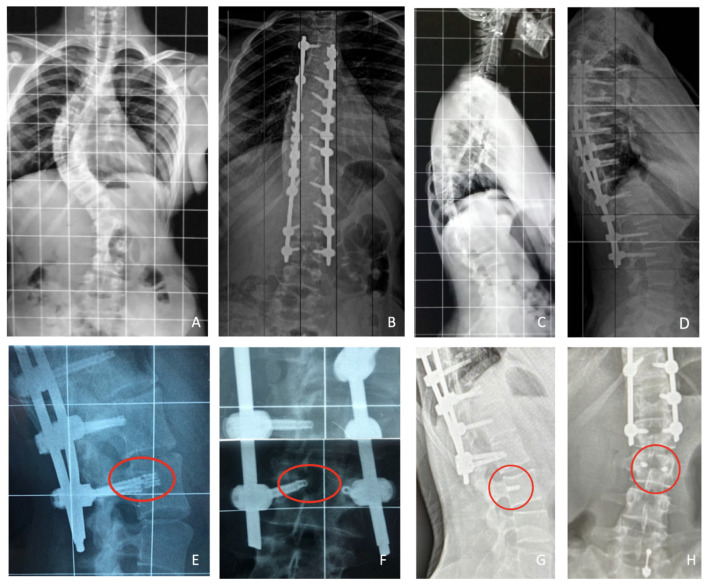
Coronal (A) and sagittal (C) standing X-rays of a 13-year-old AIS patient who underwent posterior T3–L3 lumbar fusion with FPSs (B,D). At 12 months clinical follow-up, she complained of severe back pain, and the radiographic examination showed a rupture of the screw tip in L3 as shown in the red circles (E,F). Therefore, it was decided to remove the FPSs from L3. However, as shown (G,H), it was not possible to remove the screw tip completely. At the last follow-up, two years after surgery, the patient was in good health and no changes were reported in sagittal and coronal radiographic parameters.
